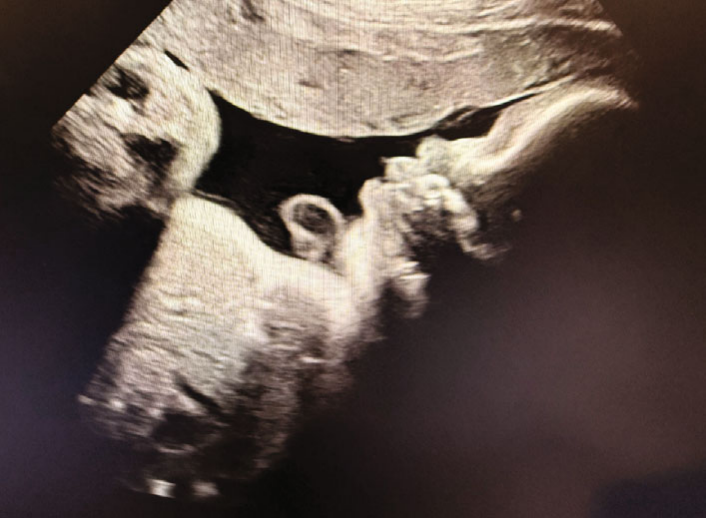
Image findings. 2D ultrasound of the fetus at 30 weeks gestation showing resolution of anterior neck mass and resolution of neck hyperextension.
